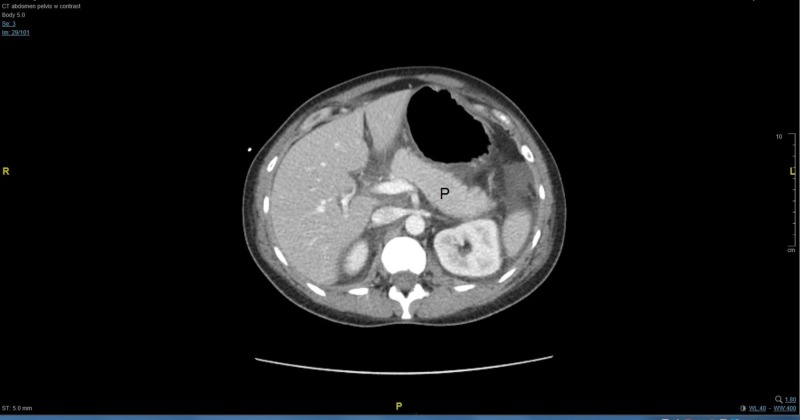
Abdominal computed tomography (CT) scan with contrast highlighting the pancreas P:pancreas
